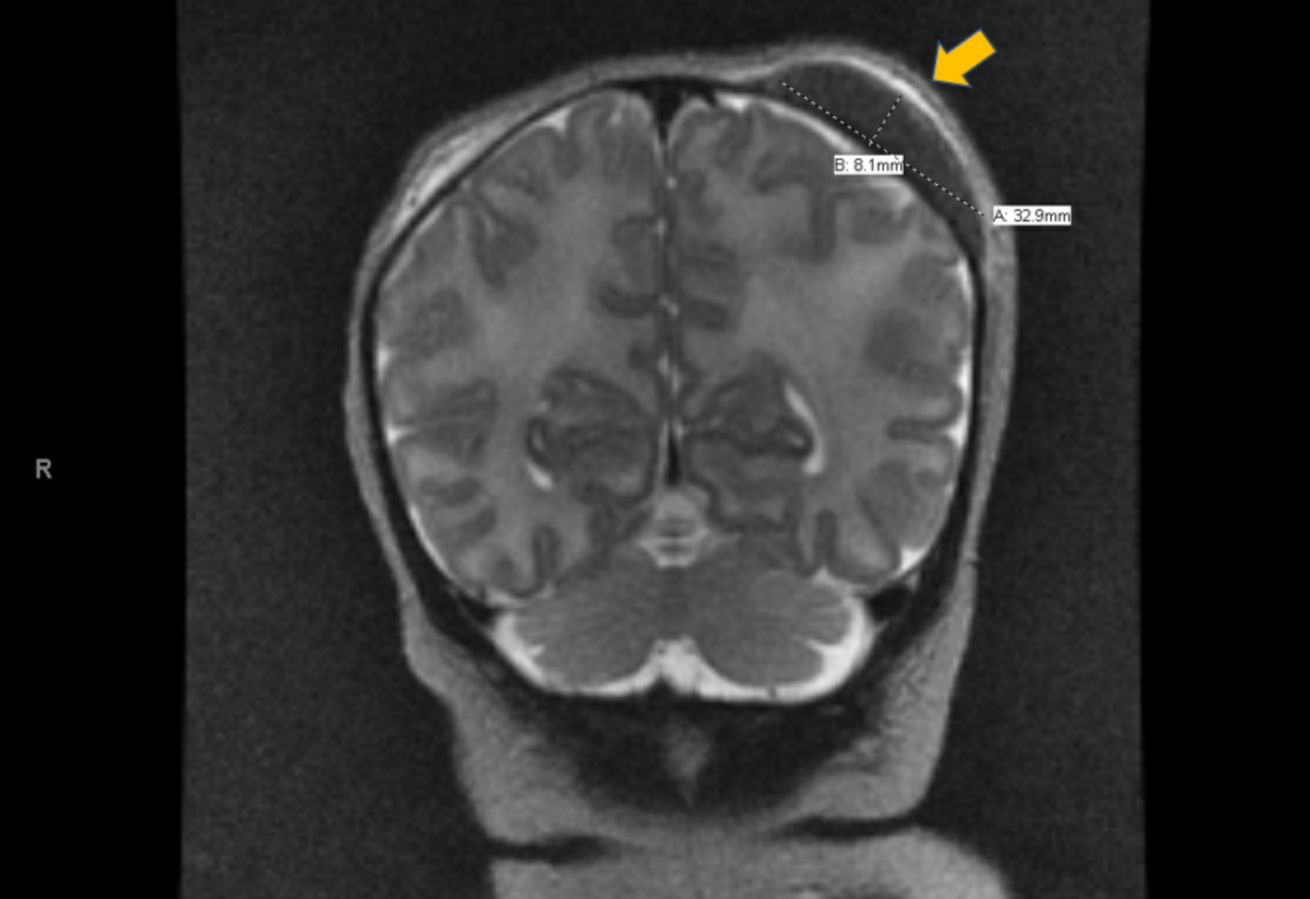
Non-contrast MRI: Coronal View A non-contrast MRI in coronal view showing the presence of a cephalohematoma with no intracranial lesion identified.
